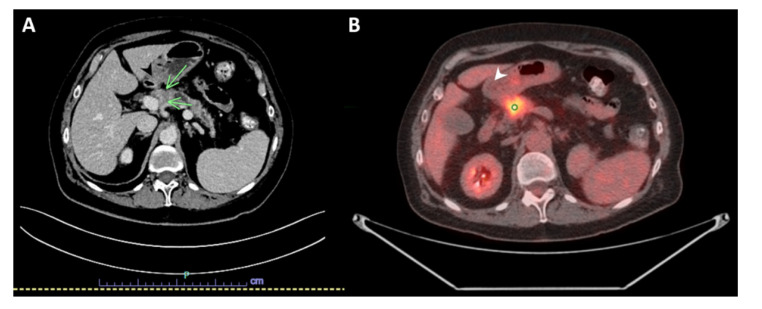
Imaging studies. (A) Computed tomography (CT) scan with IV contrast of the abdomen and pelvis showing a hypoenhancing pancreatic mass centered in the pancreatic neck, measuring 2.7 × 2 cm (green arrows). (B) Positron emission tomography (PET)/CT scan of the abdomen demonstrating mildly enlarged pancreas with a 2.7 cm heterogeneous, hypoechoic mass in the head of the pancreas with abnormal hypermetabolic uptake in the SUV MAX 9.3 (white arrow).
